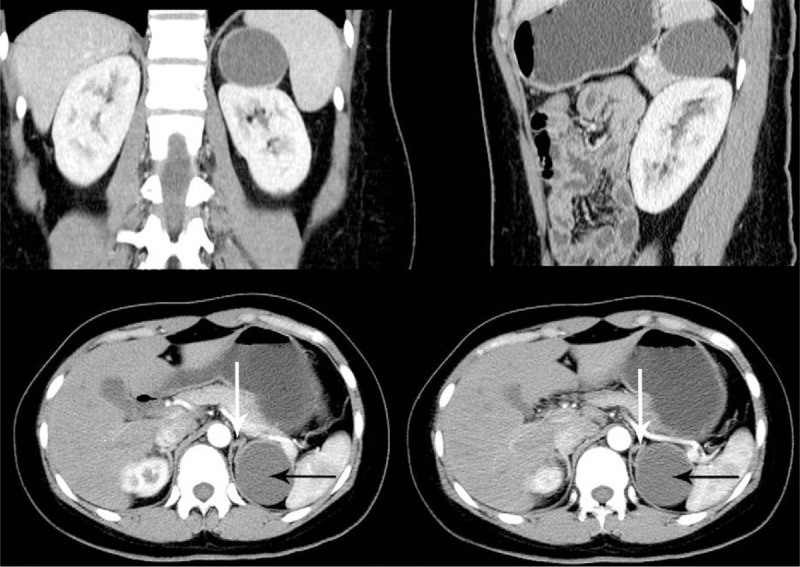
Abdominal computed tomography scan, coronal and sagittal imaging showed the cyst (black arrows), measuring 5 × 5 cm, was located at the superomedial side of kidney closely connected with adrenal gland (white arrows) without abnormal renal morphology. No reinforcement was observed after contrast-enhanced CT scanning.
